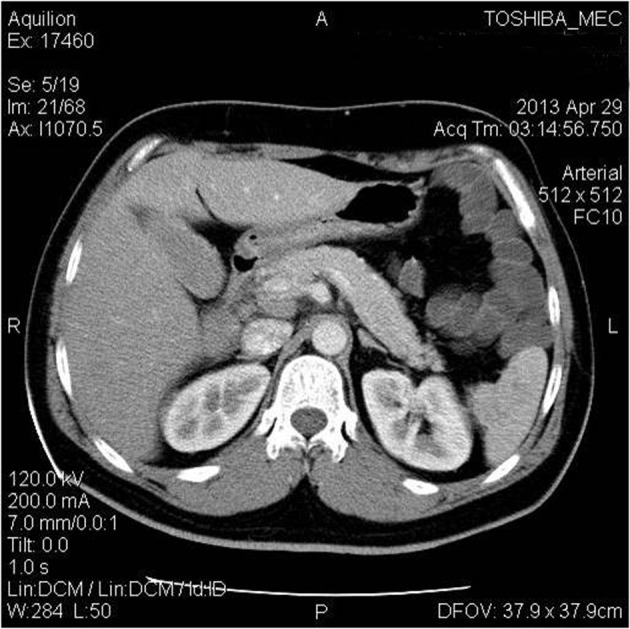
Abdominal computed tomography showing resolution of the gall bladder hematoma.
